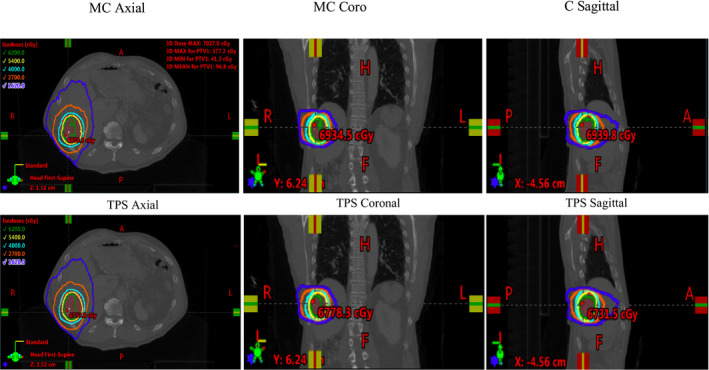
Dose distribution comparison between Monte Carlo and RayStation treatment planning system on a SABR liver patient computed tomography dataset for DWA plan.
